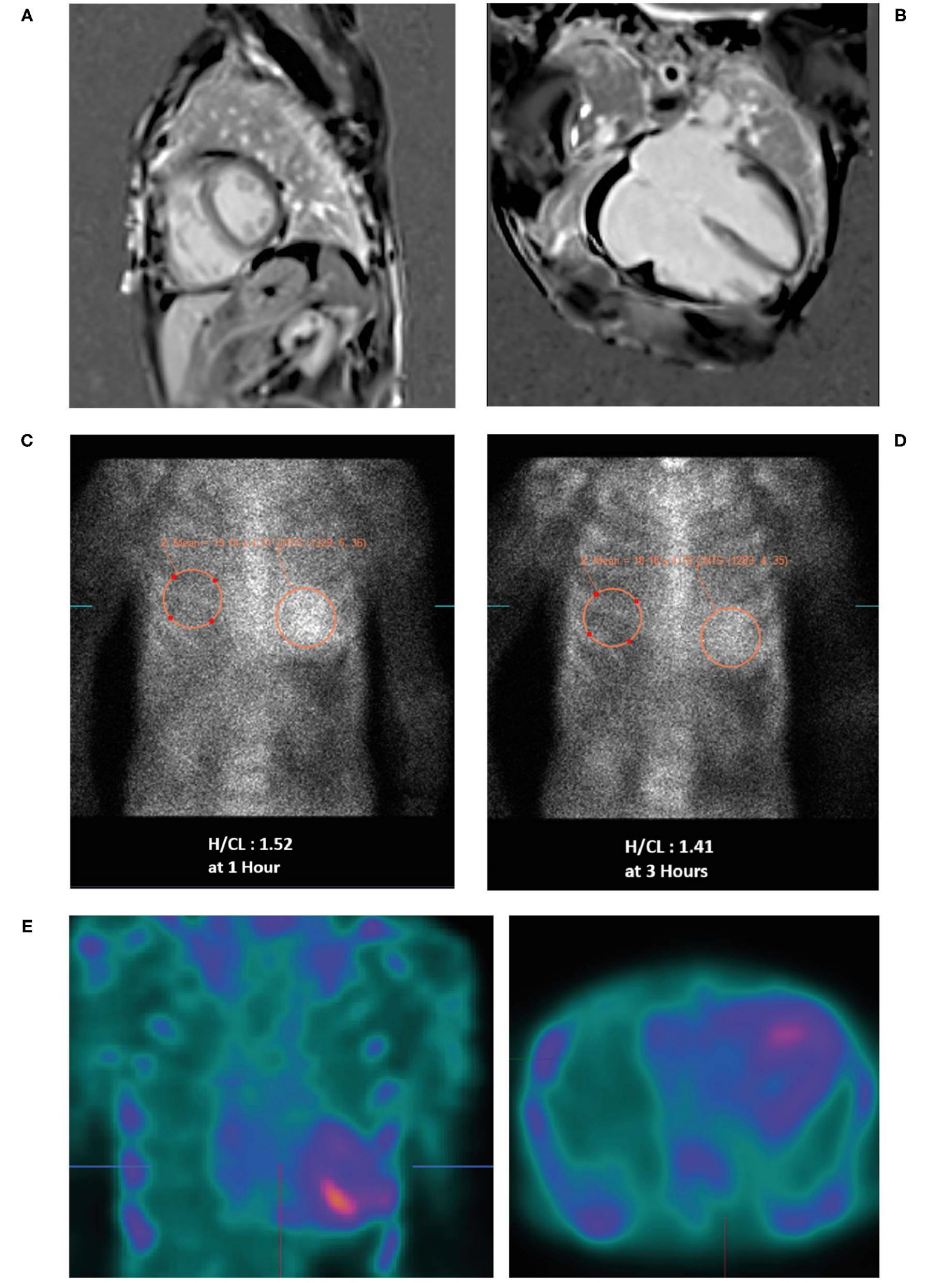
Cardiac MRI (A,B) showing biatrial enlargement without evidence of infiltrative disease. Planar bone scintigraphy (^99m^Tc-PYP) at 1 h (C) and 3 h (D) showing myocardial PYP uptake greater than rib uptake (grade 3) via semi-quantitative assessment—strongly suggestive of ATTR-CM. SPECT scan (E) at 1 h showing no blood pool. ^99m^Tc-PYP, ^99m^technetium-labeled pyrophosphate; ATTR-CM, transthyretin amyloid cardiomyopathy; MRI, magnetic resonance imaging; PYP, pyrophosphate; SPECT, single-photon emission computed tomography.
